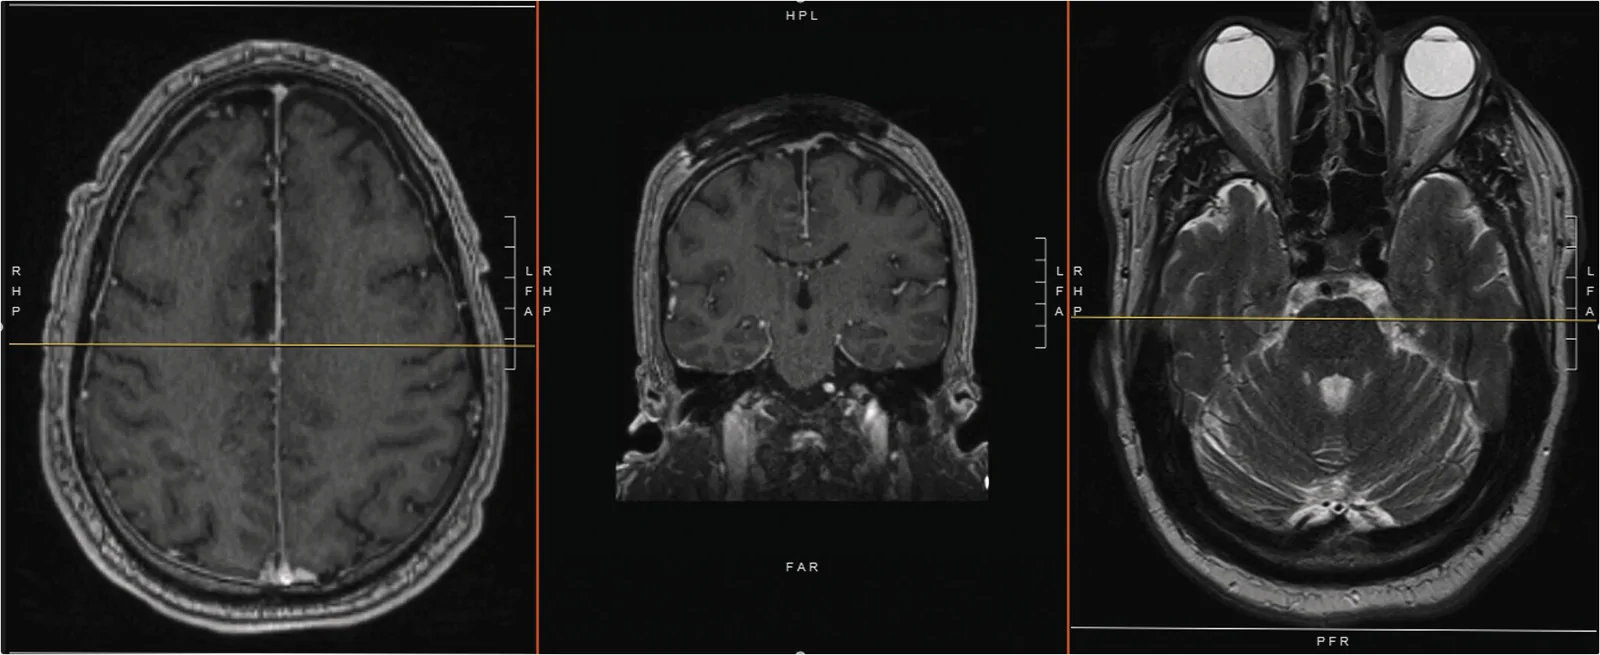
Postoperative MRI showing gross total resection.
